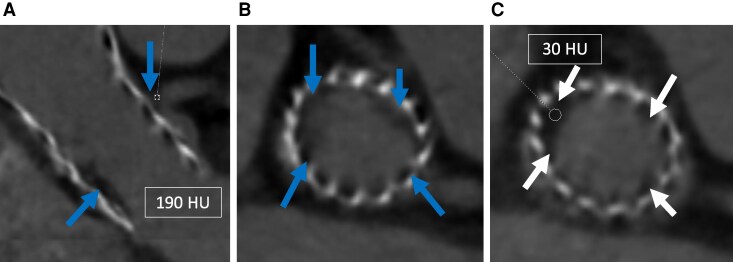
Cardiac-CT. (*A*, *B*) Circumferential pannus extending along the prosthesis up to the LM (190 HU, blue arrows). (*C*) HALT at the THV leaflet bases (30 HU, white arrows).
